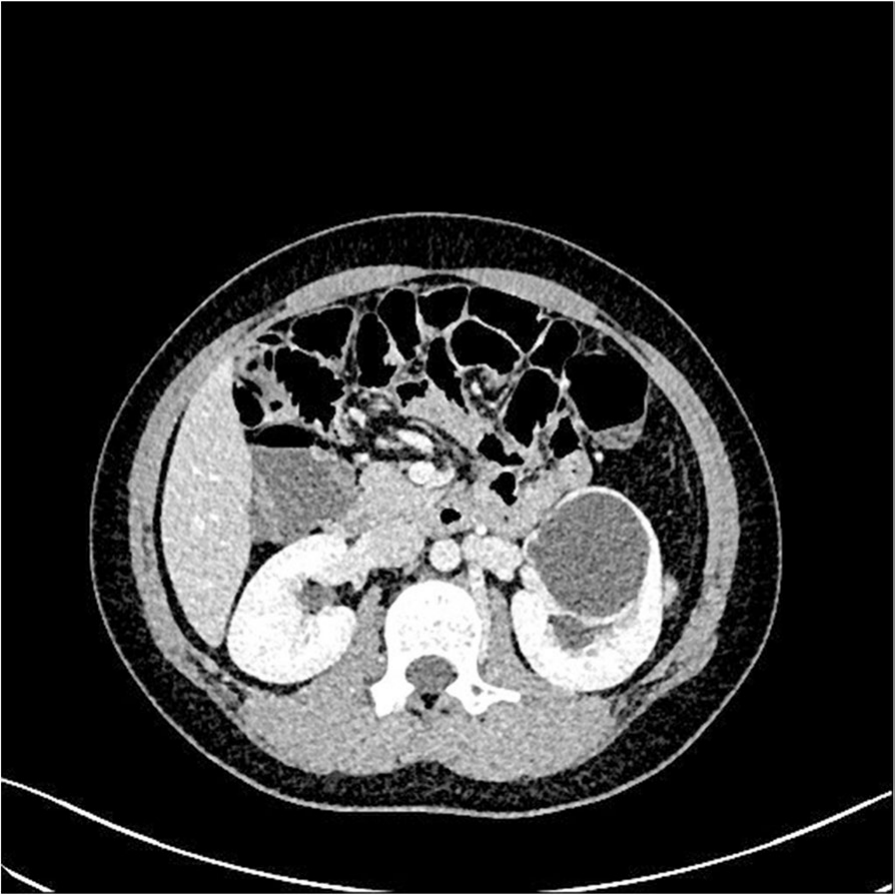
Preoperative urological CT. A rounded hypointense shadow (approximately 47.2 × 46.1 × 59.3 mm) with clear borders observed in the left kidney
